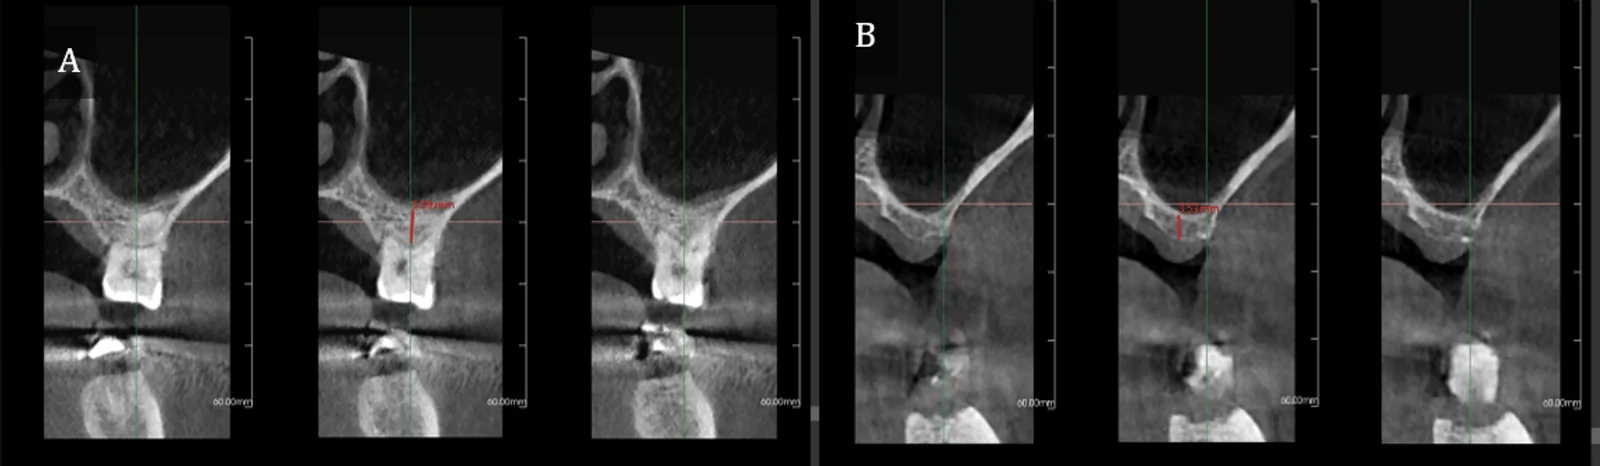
Position of maxillary sinus floor from the ridge. (A) Dentulous. (B) Edentulous. Representative CBCT cross-sectional images demonstrating the measurement of the vertical distance between the maxillary sinus floor and the alveolar ridge. (A) Dentulous maxilla, with the measurement taken from the sinus floor to the furcation of the first molar. (B) Edentulous maxilla, with the measurement taken from the sinus floor to the crest of the residual alveolar ridge. Red markings indicate the measured distance.
